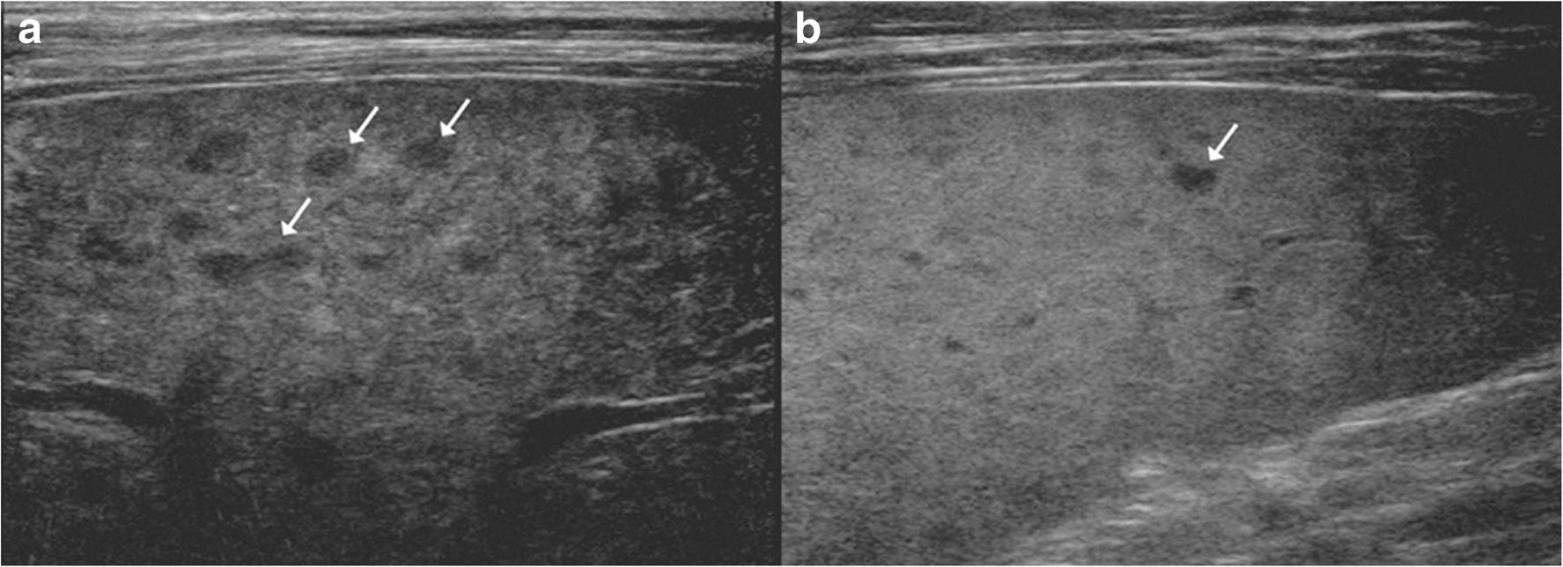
A 46-year-old man with disseminated tuberculosis and elevated liver enzymes. a, b Ultrasound images show multiple hypoechoic lesions in the liver and spleen (*arrows*) in keeping with granulomas
